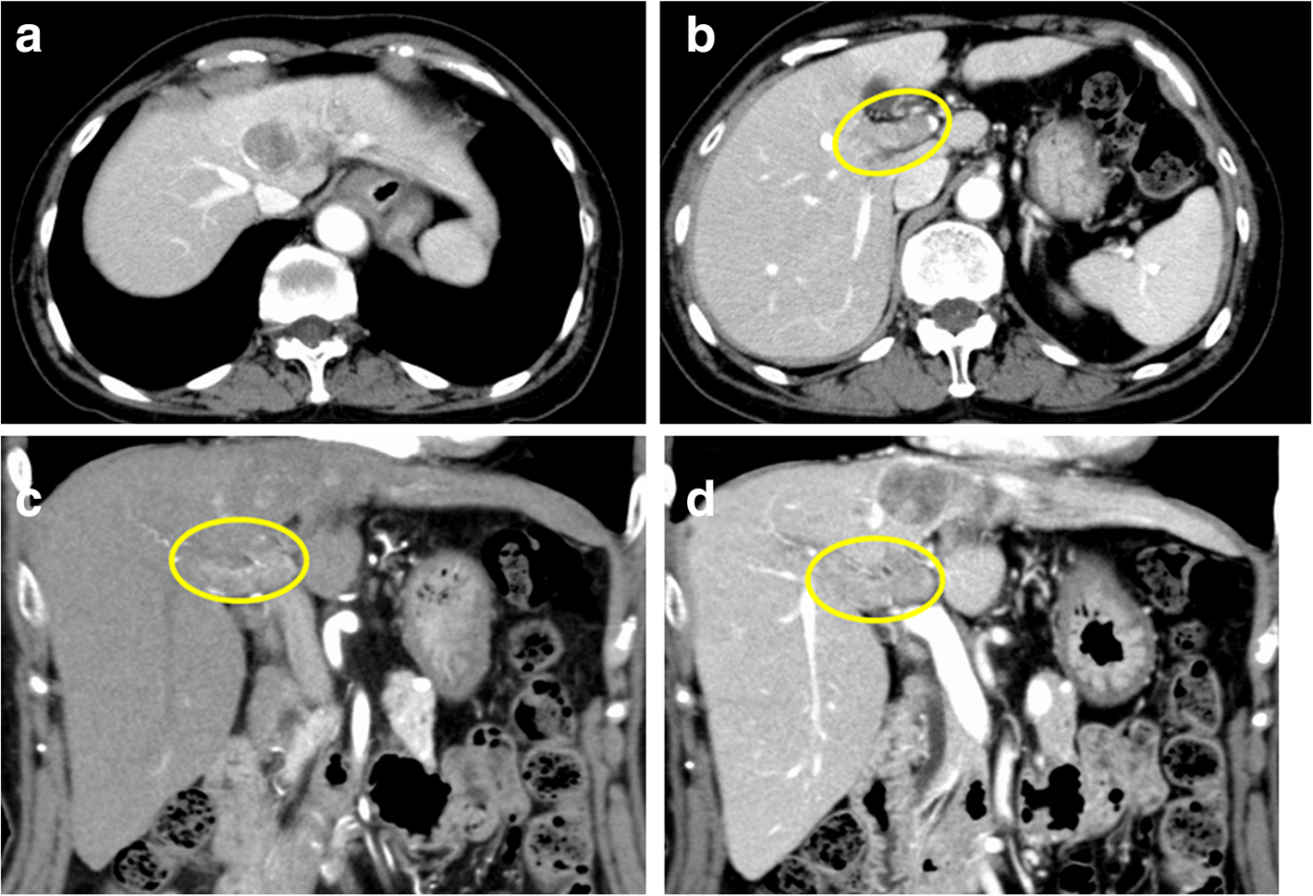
Contrast-enhanced CT before preoperative PIHP: CT showing a 28 × 25 mm HCC in segment 4 (a) and PVTT (*yellow circle*) in the left portal branch reaching the right portal (b). PVTT was enhanced in the arterial phase (c) and washed out in the equilibrium phase (d)
